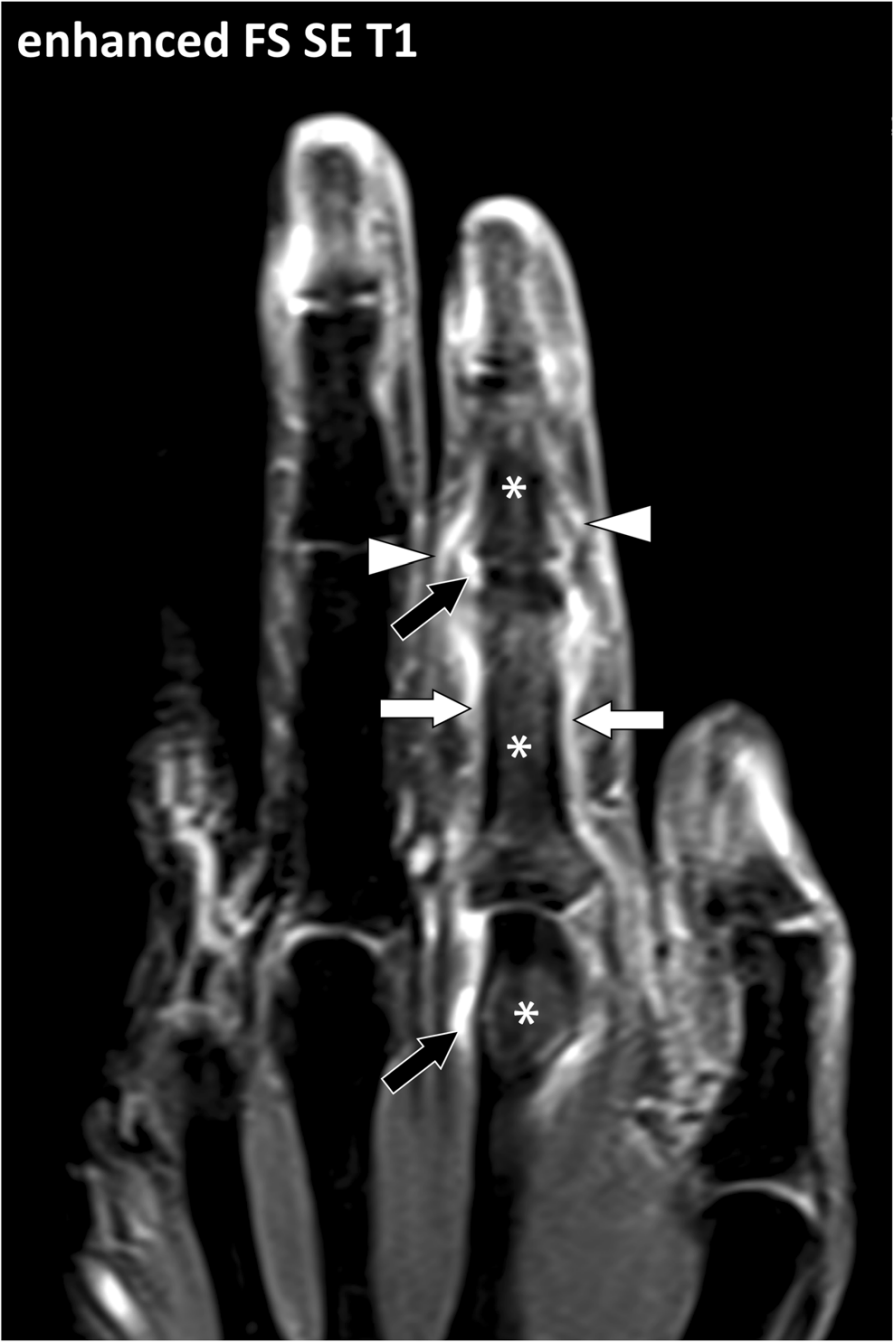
Contrast-enhanced fat-suppressed T1-weighted coronal image of the hand of a 35-year-old male with psoriatic arthritis and dactylitis of the second digit. MRI demonstrates periostitis of the proximal phalangeal bone with thickening and enhancement of the periosteum (white arrows) and osteitis (asterisks). These changes are associated with synovitis of the metacarpophalangeal and proximal interphalangeal joints (black arrows). The connective tissue located at the interfaces between tendons, joint capsules and bone is also involved (arrowheads)
